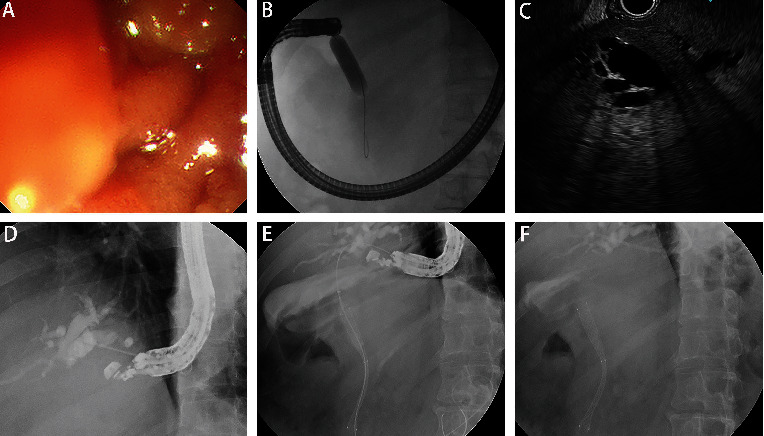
The procedure of antegrade stenting: (A) endoscopic view of the duodenal stricture; (B) X-ray image of stricture dilation with endoscope difficult to pass through subsequently; (C) endoscopic ultrasound-guided bile duct puncture pathway; (D) cholangiogram through endoscopic ultrasound-guided bile duct puncture; (E) X-ray image of released metal stent; (F) X-ray image of released metal stent with endoscopic nasobiliary drainage.
